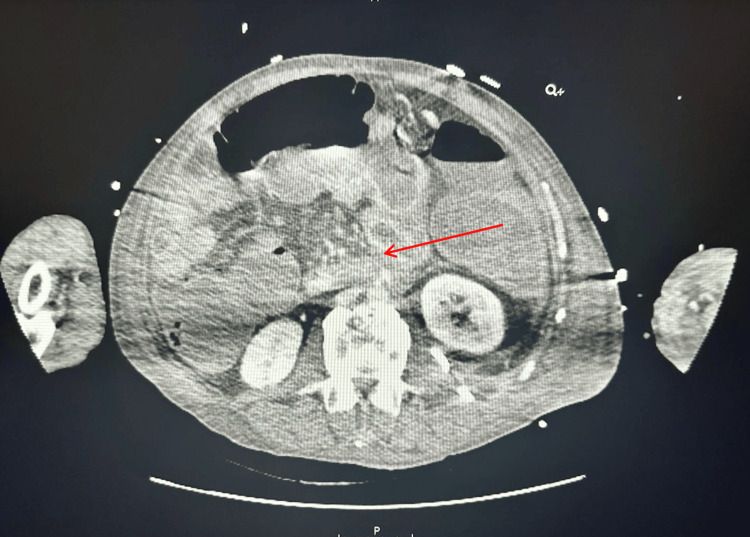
CT of the abdomen and pelvis demonstrating pooling of high-density contrast within the duodenum, which was suspicious for active hemorrhage within or near the duodenum (red arrow) CT: computed tomography
